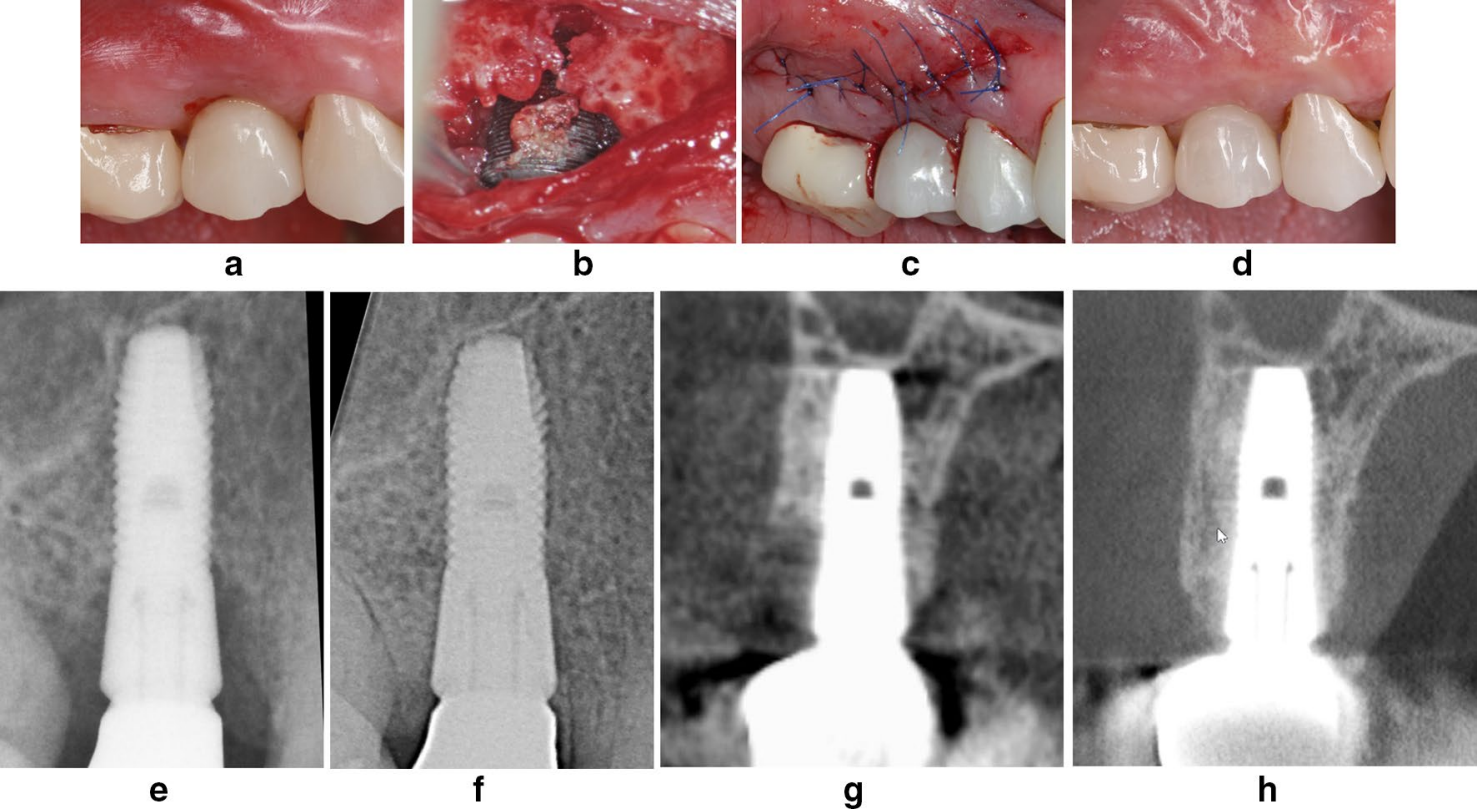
a Severe peri-implantitis lesion with suppuration and bleeding on probing. b Access to the severe buccal peri-implant bony lesion by the LAPIDER approach. c Hard and soft tissue defect grafting and bilaminar wound closure. d At 3-year follow-up examination no signs of a peri-implant infection are left. e The baseline radiograph reveals a severe interproximal bone loss. f A radiograph at 2-year follow-up examination shows the complete interproximal regeneration. g The pre-operative CB-CT shows a severe buccal bone loss. h At 3-year follow-up a CB-CT reveals the complete bone regeneration to the implant shoulder level
